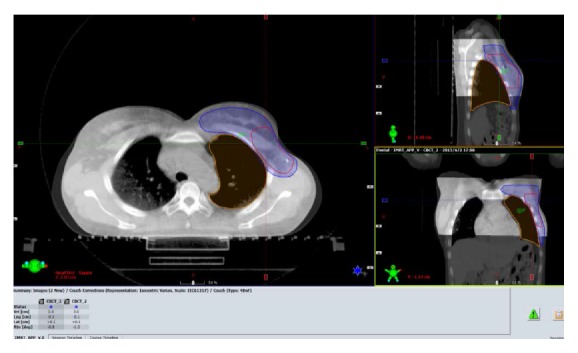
The cone-beam computed tomography (CBCT) registration of the treatment areas. The table below the image shows the displacement after the CBCT registration.
